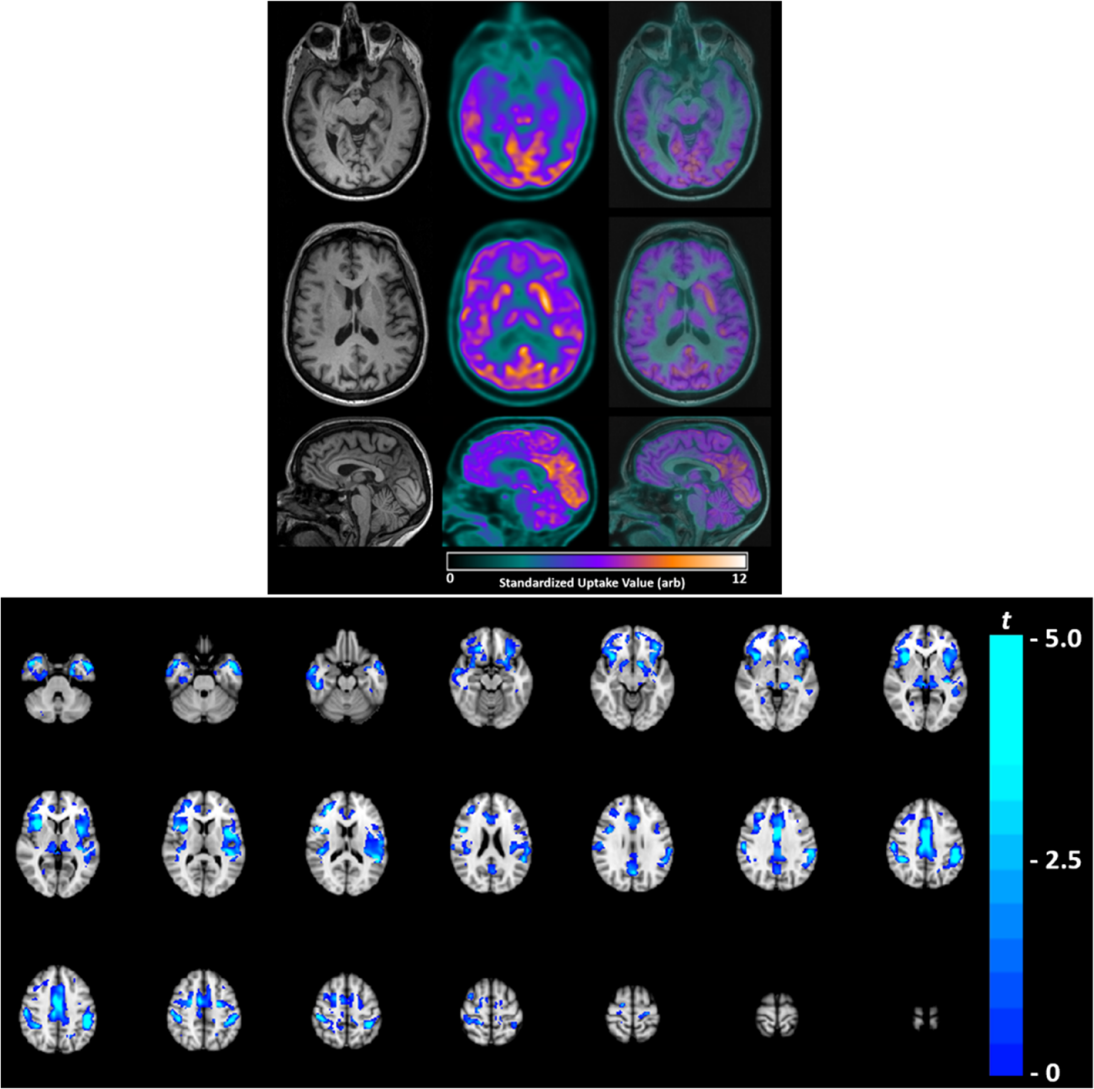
Top section - Axial and sagittal slices of the patient’s brain acquired on a PET/MRI; T1-weighted anatomical (left), FDG-PET (middle) and FDG-PET overlaid onto T1-weighted (right). No significant brain atrophy was detected on volumetric measurement of T1-weighted images. Decreased FDGuptake was visible in the frontal and temporal regions of the brain. Bottom section - FDG-PET t-map (t > 2, *p* < 0.05) overlaid to the standard MNI T1-weighted image. Areas of hypometabolism (blue-green) were observed in medial superior frontal, insula, inferior temporal, thalamus, and anterior cingulate cortex
